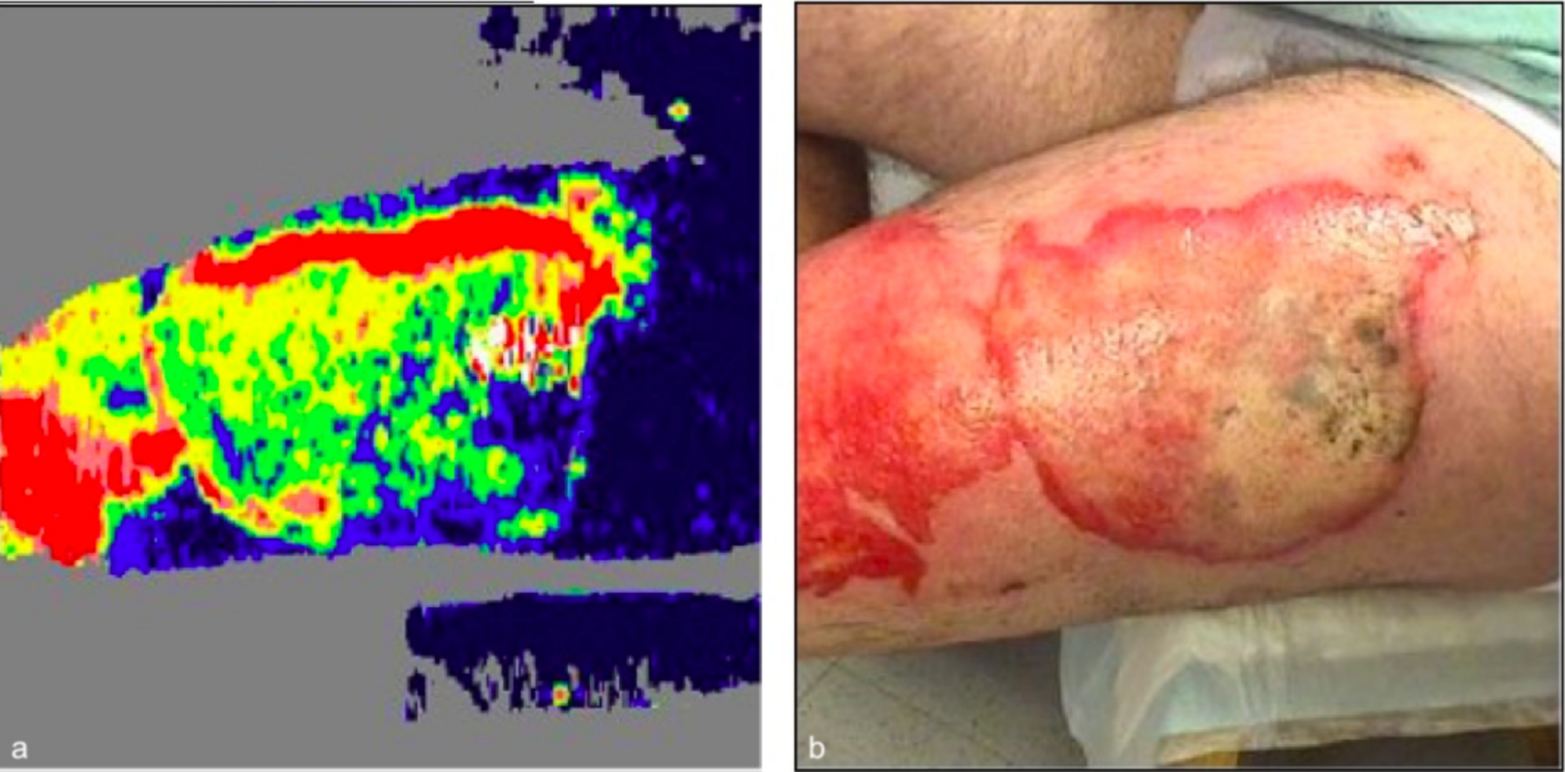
Laser Doppler Imaging (LDI) performed 48 hours post-injury on the lateral aspect of the left thigh. LDI (a) and photo (b).
